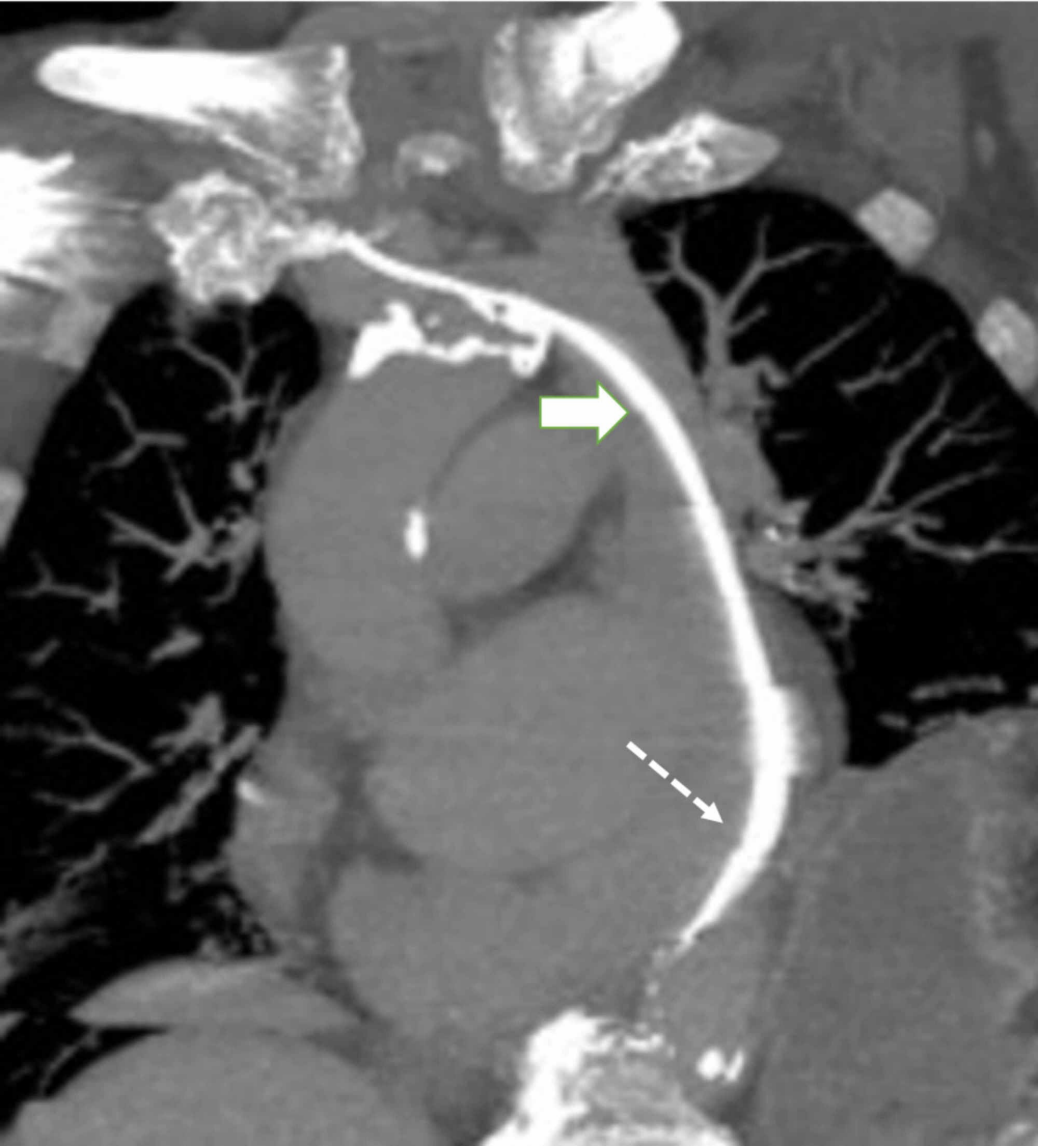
CT of the chest with subtraction imaging demonstrating the course of pacer lead through persistent LSVC (solid arrow) and CS (dotted arrow) CS: coronary sinus, LSVC: left superior vena cava
